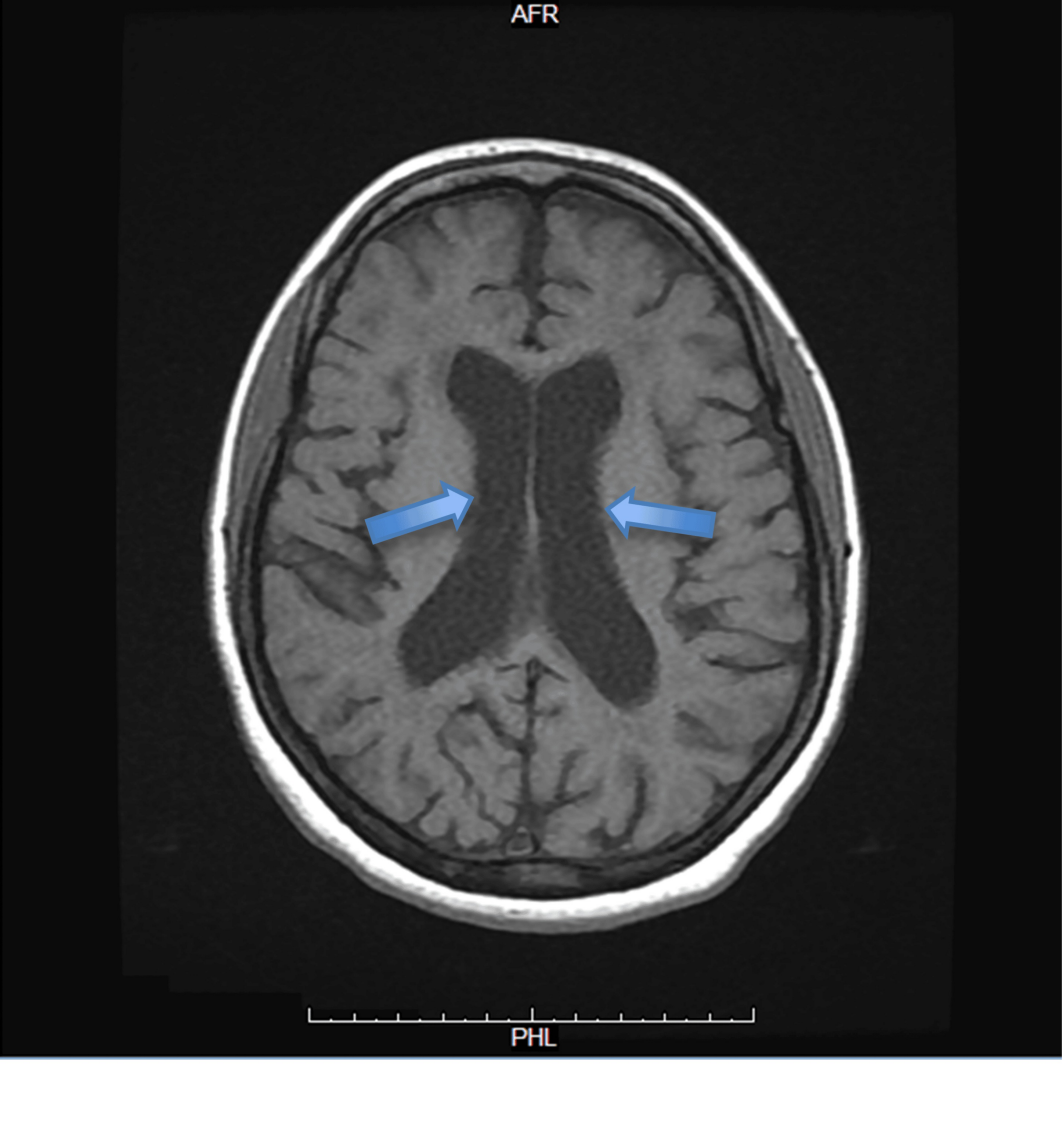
Axial T1-weighted MRI of the brain without contrast This axial T1-weighted MRI demonstrates prominent lateral ventricles (blue arrows) and diffuse cortical sulcal widening, indicating cortical atrophy disproportionate to patient age. The periventricular and deep white matter appear hypointense (darker on T1) MRI: magnetic resonance imaging
